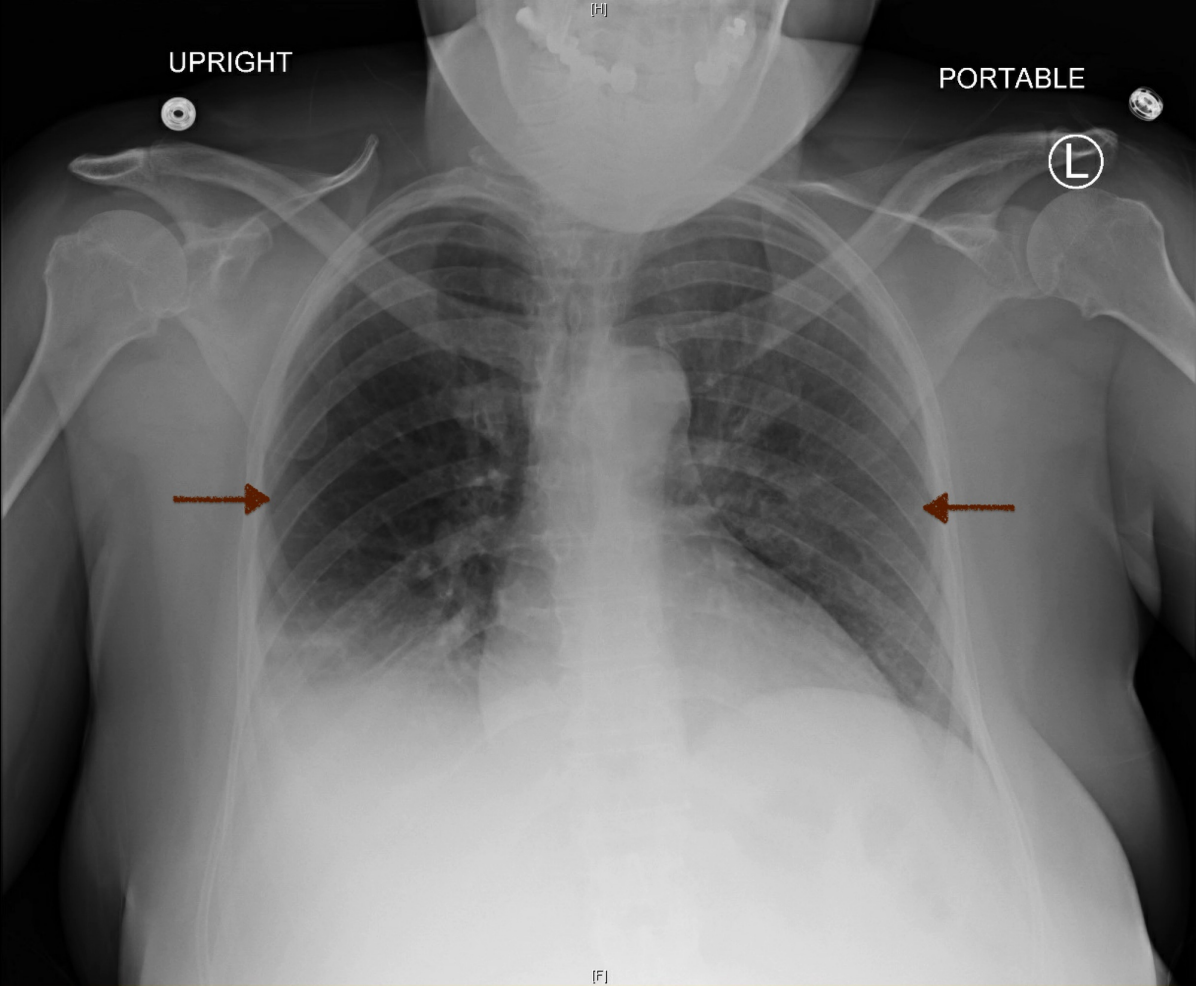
Chest X-Ray Continued improvement of the bilateral opacities (red arrows). L: Left. H: Head. F: Feet.
